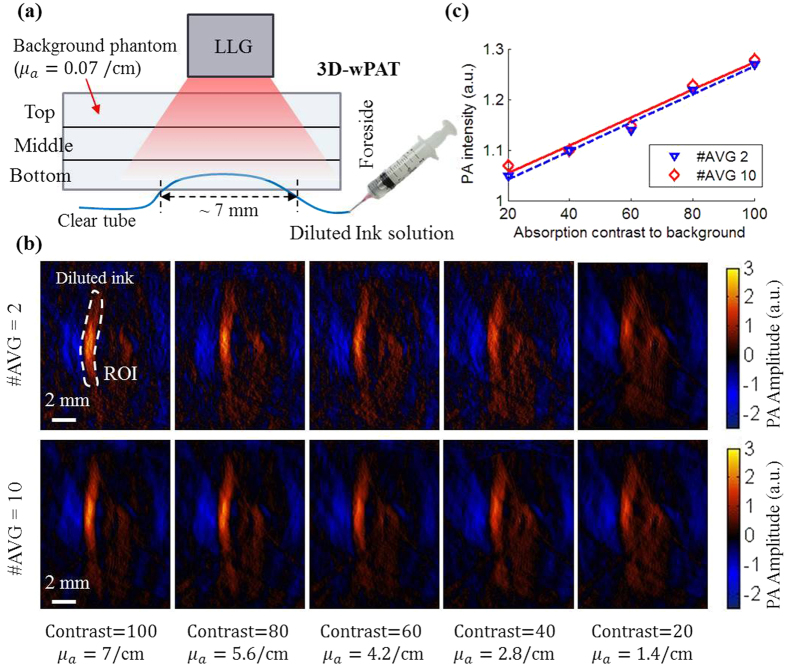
(a) Experimental setup. (b) wPAT image obtained by the bottom ultrasound detection layer. (c) Mean PA intensity of the ROI with respect to absorption contrast and averaging times. #AVG: times of averaging.
